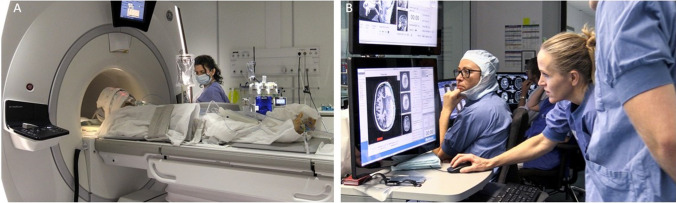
Pre-treatment MRI A The flex coil is positioned around the patient’s head for imaging acquisition. B The MRI is connected to the Visualase console. Two Tmap imaging planes, in this case axial and sagittal, are selected for continuous thermometry imaging during treatment
